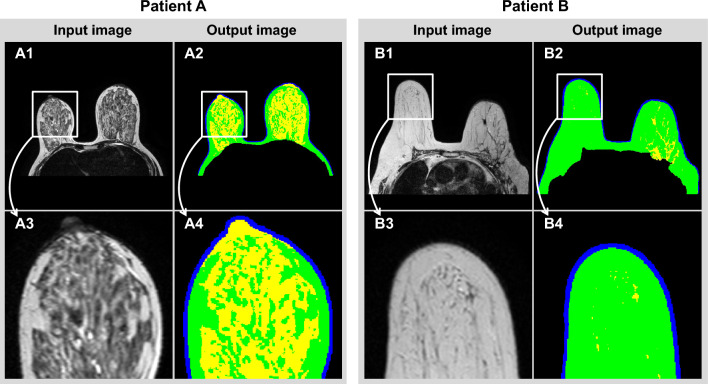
MRI images and breast tissue segmentation images of Patient A with a high segmentation accuracy (mIoU: 90.28) and Patient B with a low segmentation accuracy (mIoU: 77.94).
